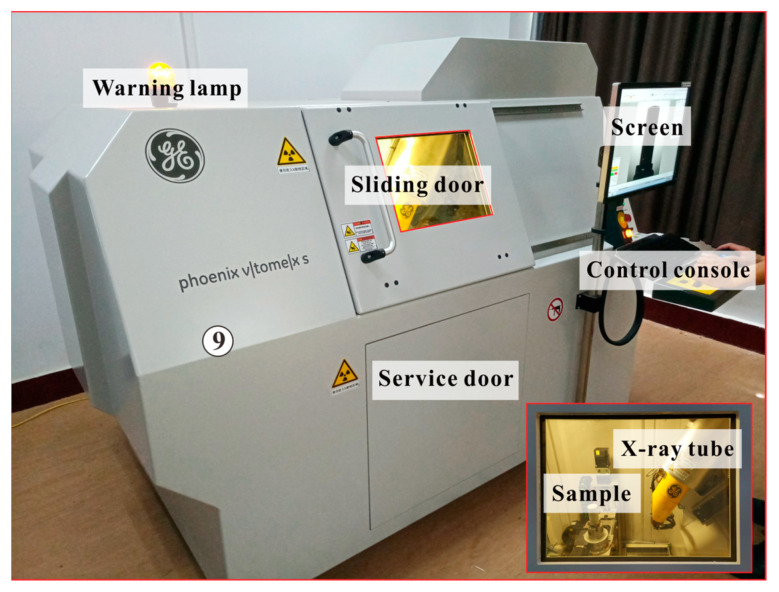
Apparatus for computed tomography (CT) test.
